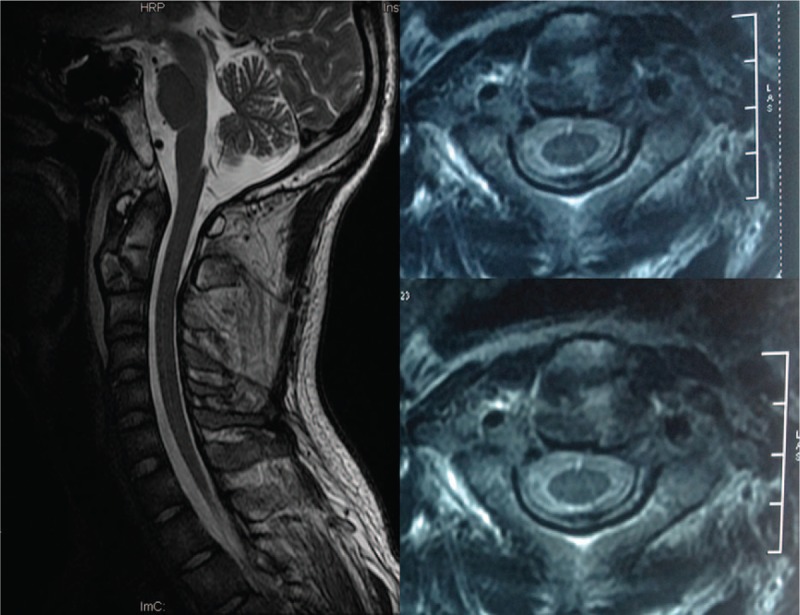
Preoperative cervical MRI showed hyperintensity in C2/3 intervertebral disc and pre-cervical soft tissue. MRI = magnetic resonance imaging.
